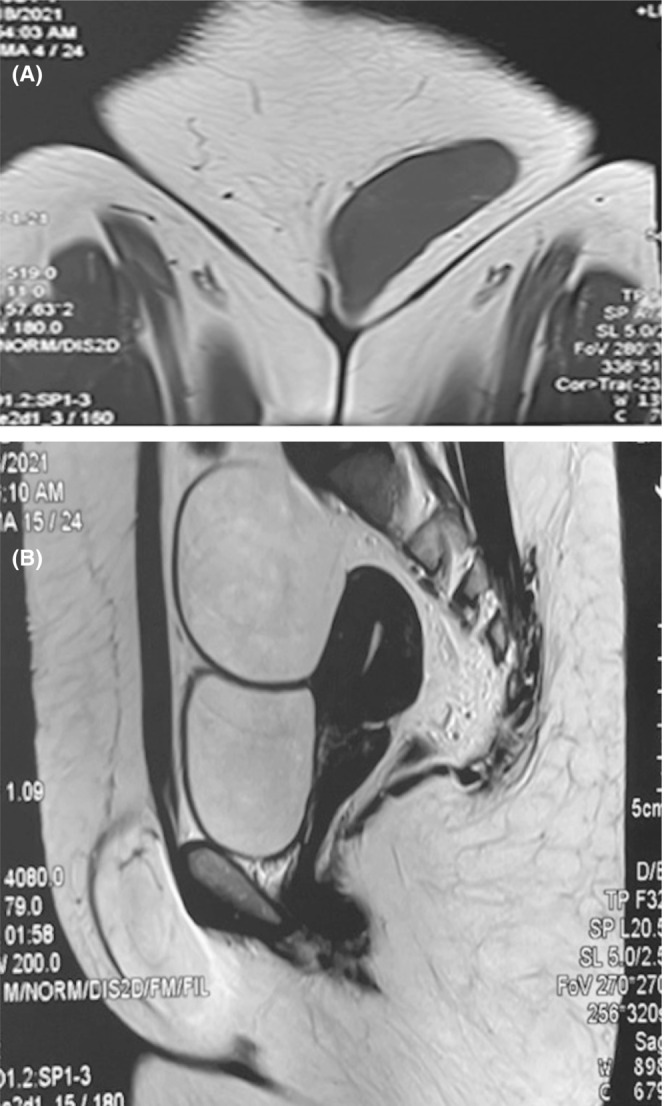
MRI showing well‐encapsulated cystic lesion in the left inguinal region, hyperintense on T1, profoundly hyperintense on T2.
